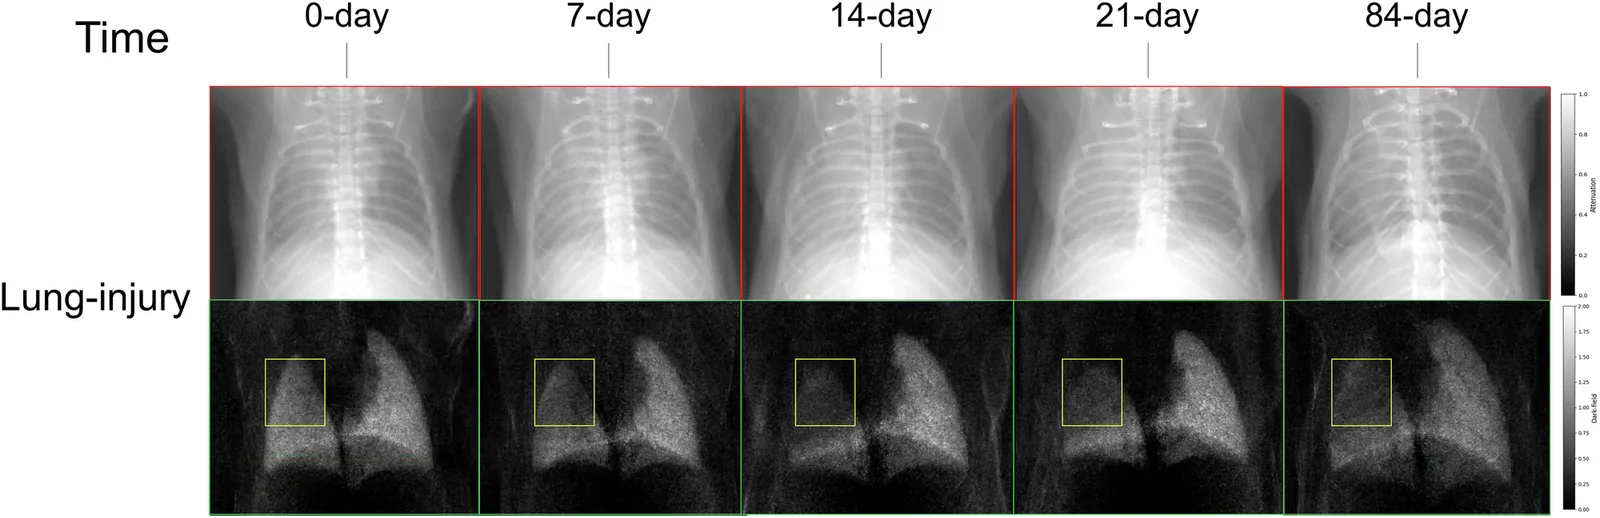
Representative longitudinal digital radiography images of a lung injury model
